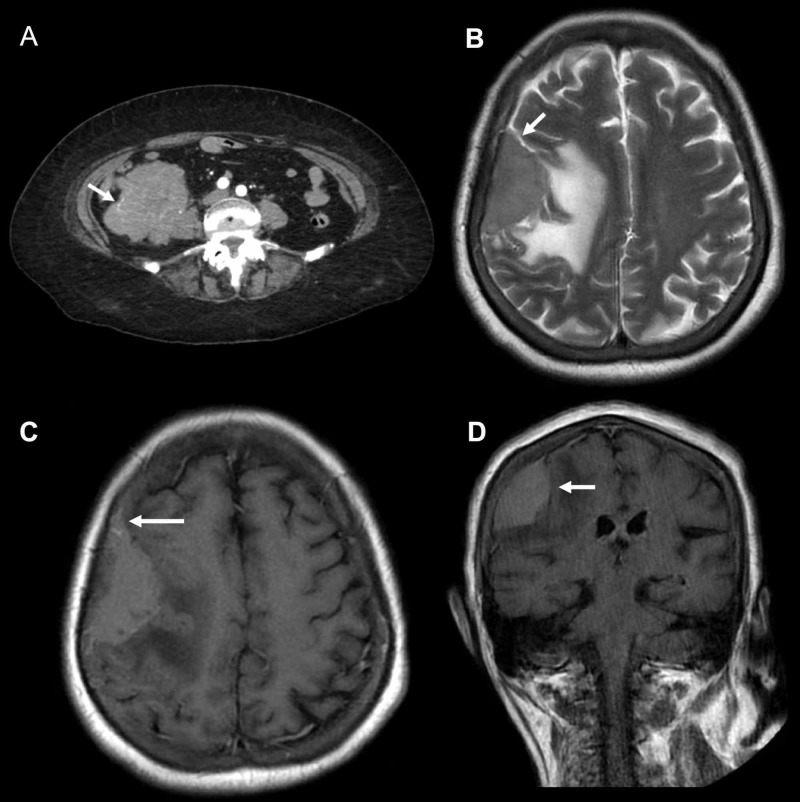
Abdominal computed tomography (CT) and brain magnetic resonance imaging (MRI) findings (A) Abdominal CT shows a contrast-enhancing ill-defined appendicular mass; (B) Axial T2-weighted brain MRI shows an extra-axial right frontotemporal dural mass with perilesional edema and a cerebrospinal fluid cleft (arrow); (C-D) Post-contrast axial and coronal T1-weighted MRI shows a heterogeneously enhancing round mass with well-circumscribed margins and dural tail sign.
